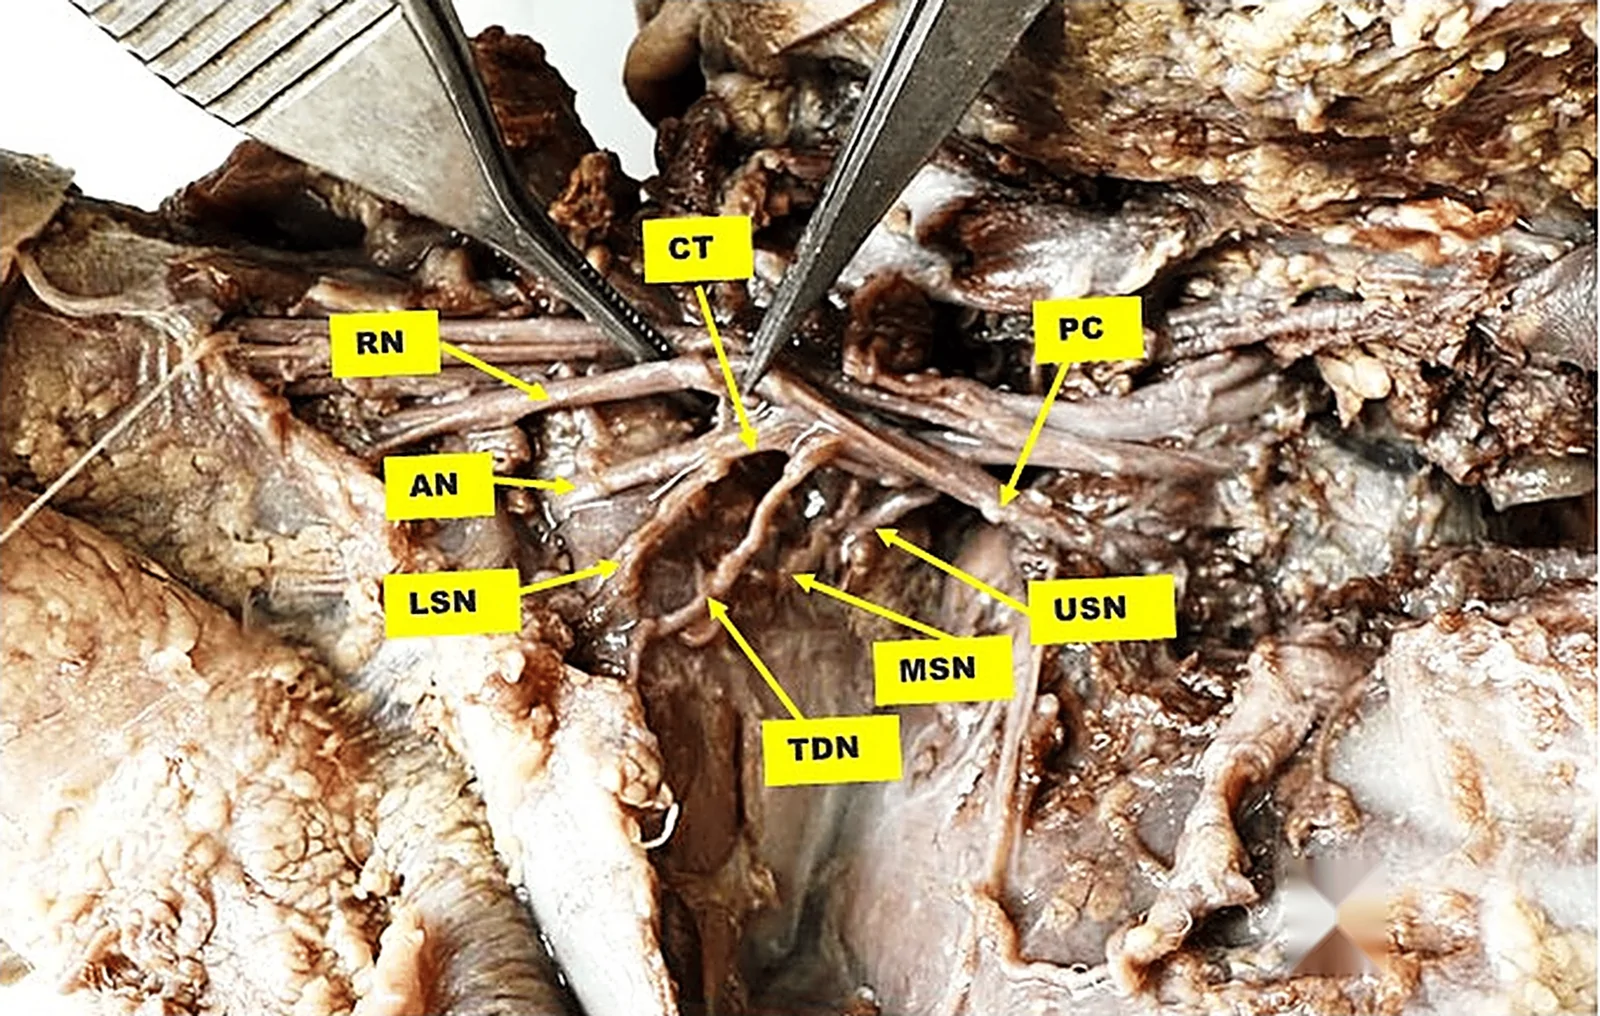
Common trunk for the axillary and lower subscapular nerves with three subscapular nerves. A common AN-LSN trunk is present together with three subscapular nerves arising from the posterior cord. AN: axillary nerve; LSN: lower subscapular nerve; TDN: thoracodorsal nerve; USN: upper subscapular nerve; MSN: middle subscapular nerve; PC: posterior cord; RN: radial nerve; CT: common trunk
